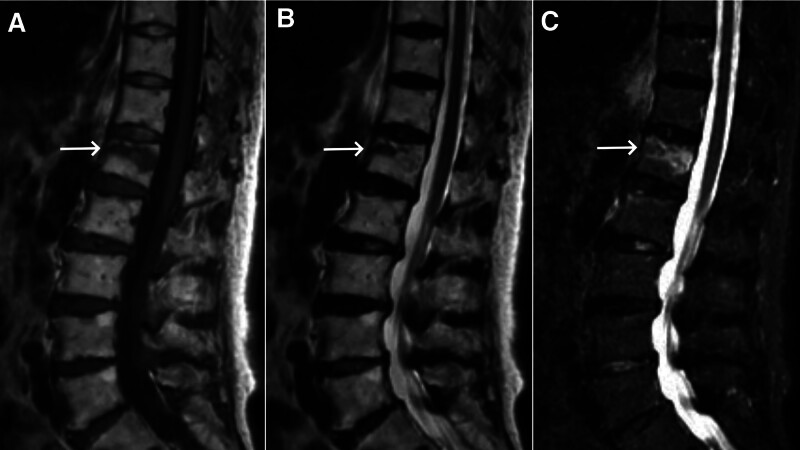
(A) Preoperative magnetic resonance imaging (MRI) in the T1-weighted phase revealed patchy, long T1 signal shadows (arrow) within the L1 vertebral body. (B) Preoperative MRI in the T2-weighted phase showed patchy, long T2 signal shadows (arrow) within the L1 vertebral body. (C) Preoperative MRI with fat suppression imaging showed patchy high signal (arrow) within the L1 vertebral body.
